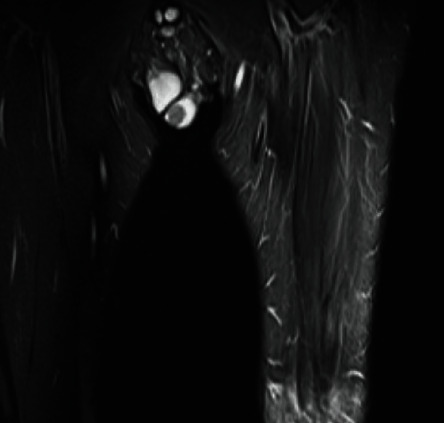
MRI with T2 imaging demonstrating hypointense nodule on the left testis.
